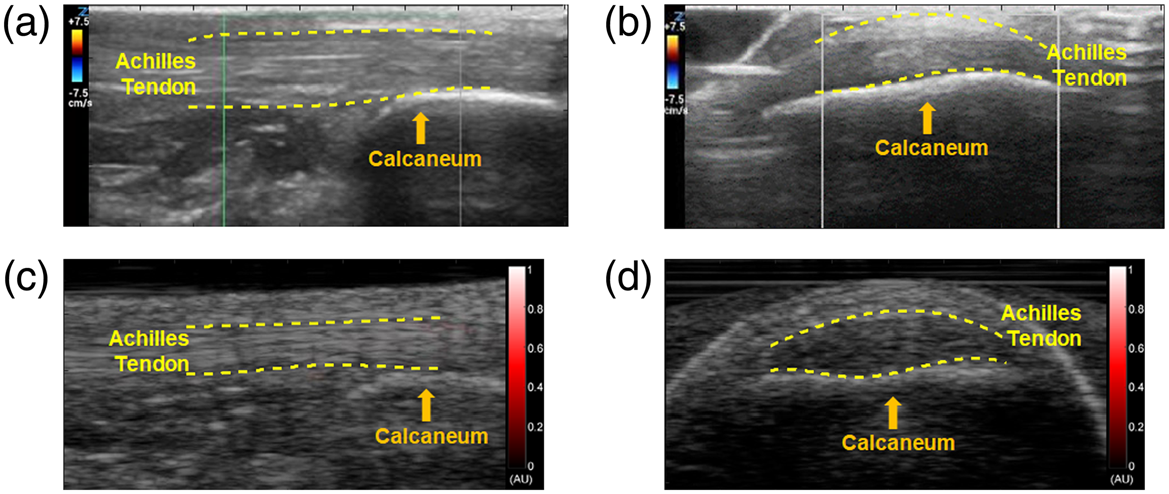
US Doppler and PA imaging of an Achilles tendon of a normal volunteer. (a) and (b) US Doppler images of the volunteer’s Achilles tendon when scanned along the long and the short axes of the tendon, respectively. (c) and (d) PA images of the volunteer’s Achilles tendon when scanned along the long and the short axes of the tendon, respectively. No hyperemia noted.
